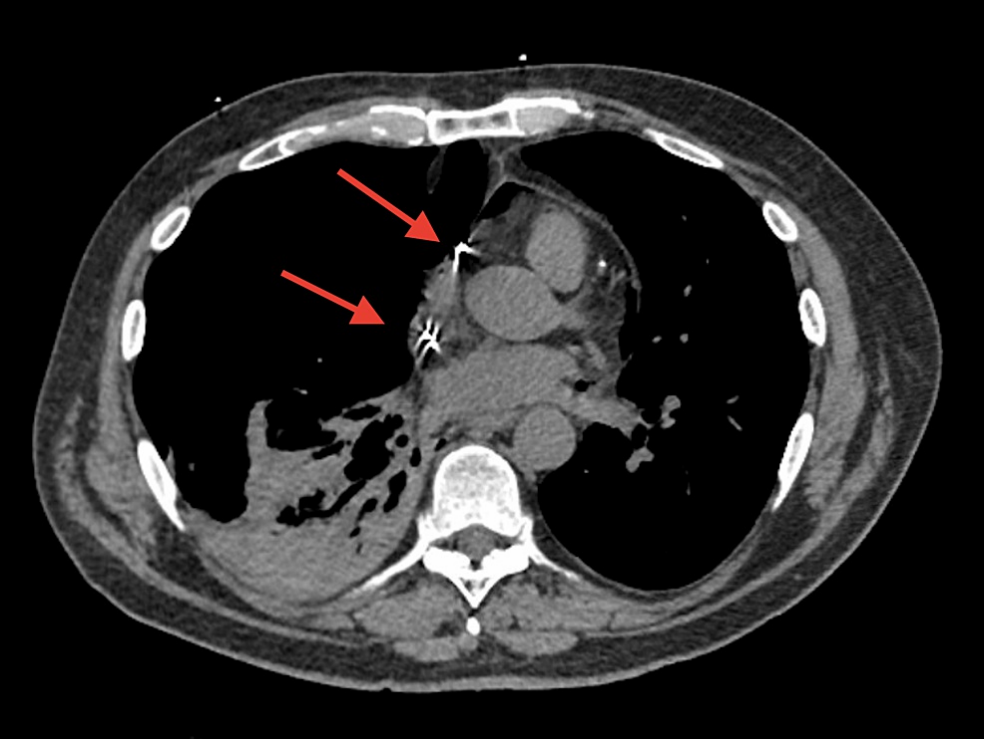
CT of the chest demonstrating tip of wires (red arrows) appears to be in the region of the right atrium, right ventricle region though the right ventricular lead appears to extend beyond the wall of the right ventricle area and it is hard to determine if it is within the wall or within pericardium. Similarly, right atrial lead also appears intended right atrium and perforation is also a possibility.
